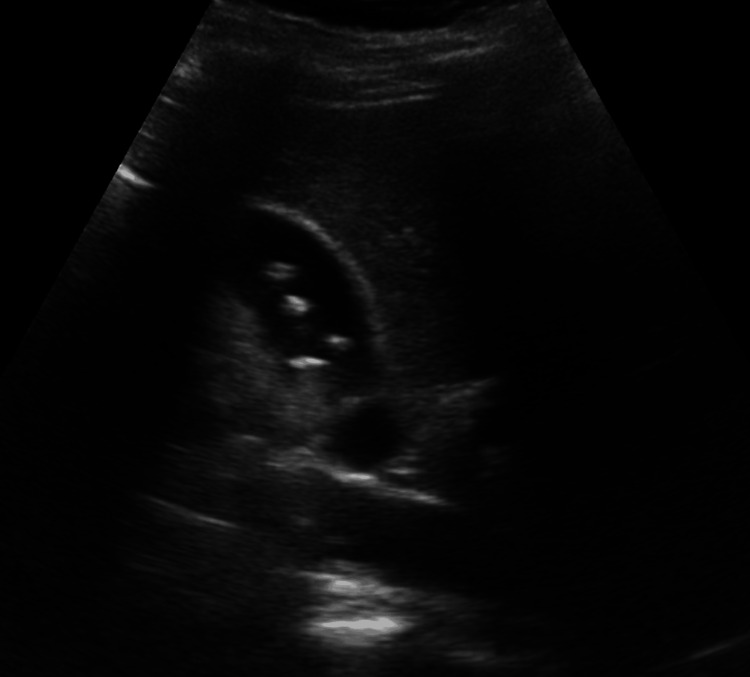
Ultrasound: multiple cholesterol gallstones (4-9 mm in size) Multiple cholesterol gallstones are observed at the level of the gallbladder without a posterior acoustic shadow. Five stones are visible in this section, with more than 10 in total.
